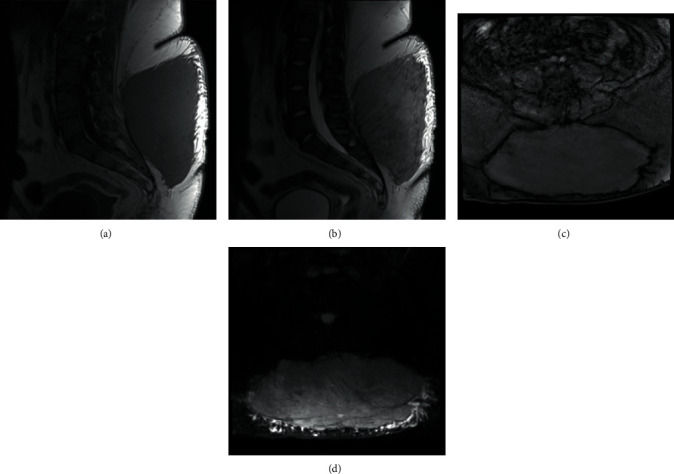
Representative preoperative 1.5 Tesla MRI images. (a) T1 TSE sagittal (TR 625 ms, TE 13 ms). (b) T2 TSE sagittal (TR 4160 ms, TE 97 ms). (c) T1 VIBE FS axial (TR 5.07, TE 2.39 ms). (d) T2 TSE FS axial (TR 5080 ms, TE 81 ms). These images revealed a large mass in the subcutaneous tissues of the lower back. The mass measured 24.8 cm × 10.4 cm × 20.5 cm in transverse, anteroposterior, and caudocranial dimensions. It extended in close proximity to the superficial fascial plane over the paraspinal musculature. No definite muscular involvement was identified. The lesion demonstrated high signal on the T2-weighted sequences and intermediate signal on the T1-weighted sequences. TSE = turbo spin echo, FS = fat suppression, VIBE = volumetric interpolated breath-hold examination, TR = repetition time, and TE = echo time.
